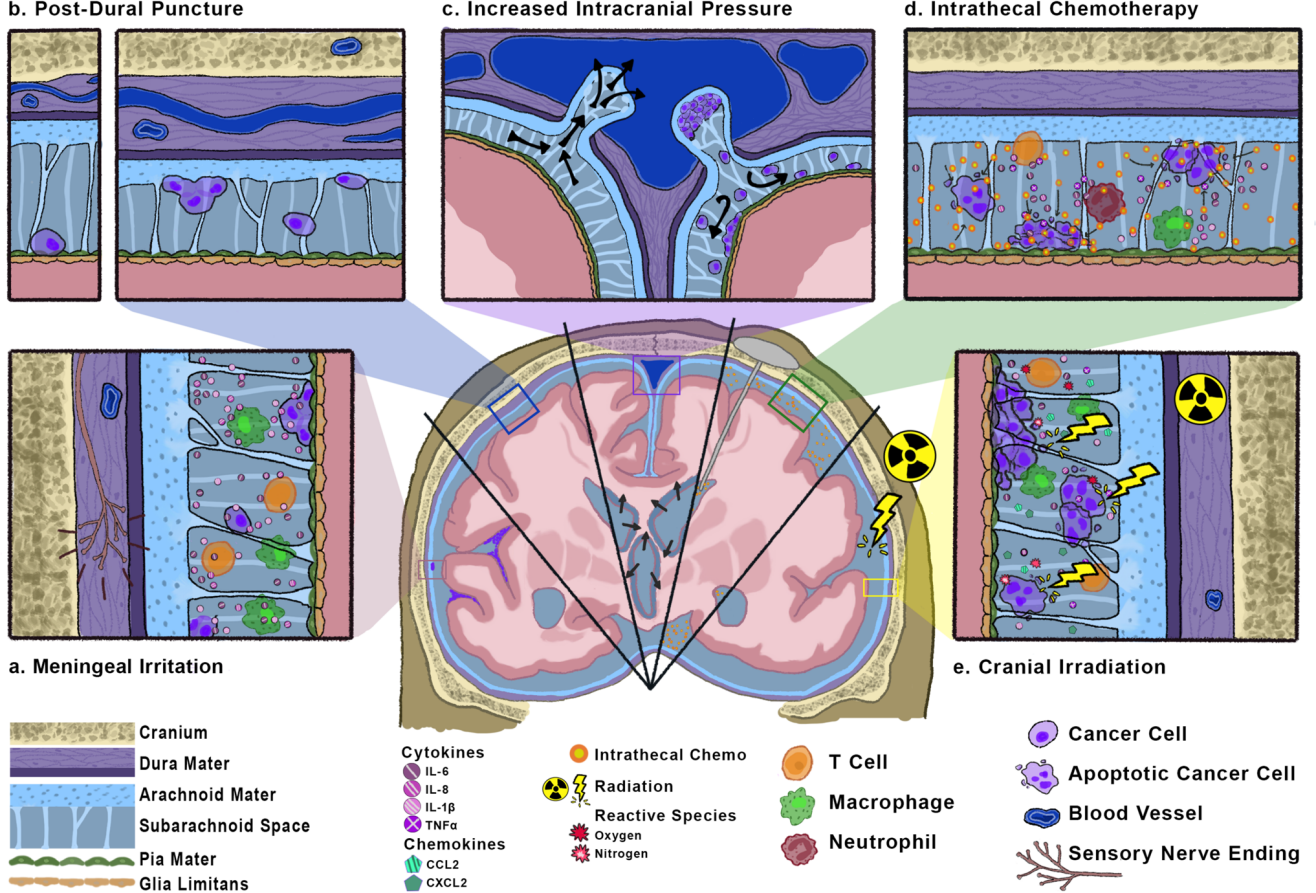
Pathophysiologic representation of five headache subtypes in patients with leptomeningeal metastases. In the setting of leptomeningeal metastases, cancer cells can be found free-floating in the CSF or adherent to the leptomeninges, transitioning between the adherent and floating states. a In disease-related meningeal irritation, sensory nerves embedded in the dura mater are theorized to experience innate-mediated activation. Myeloid cells (e.g., macrophage) and lymphoid cells (e.g., T cells) outnumber cancer cells, and higher levels of inflammatory cytokines (IL-6, IL-8, IL-1β) are observed. b Post-dural puncture headaches result from persistent CSF leakage incident to the dural hole and intracranial hypotension. On contrast-enhanced MRI brain, pachymeningeal thickening and enhancement are observed; a proposed explanation is compensatory dural vein engorgement. c Elevated intracranial pressure arises as cancer cells fill arachnoid granulations, disrupting the normal drainage of CSF from the subarachnoid space into dural venous sinuses. Brain imaging reveals communicating hydrocephalus or “ballooning” of the ventricles. d Intrathecal chemotherapy delivered via Ommaya reservoir causes a chemical arachnoiditis in the subarachnoid space, marked by increased leukocytes (T cells and macrophages), granulocytes (neutrophils), and inflammatory cytokines. e Ionizing radiation activates resident microglia, releasing pro-inflammatory cytokines (IL-6, IL-8, IL-1β, TNFα), chemokines (CCL2, CXCL2), and reactive oxygen and nitrogen species into the CSF. With increased blood-brain and blood-CSF barrier permeability, additional T lymphocytes and macrophages infiltrate the subarachnoid space and add to the inflammatory milieu
